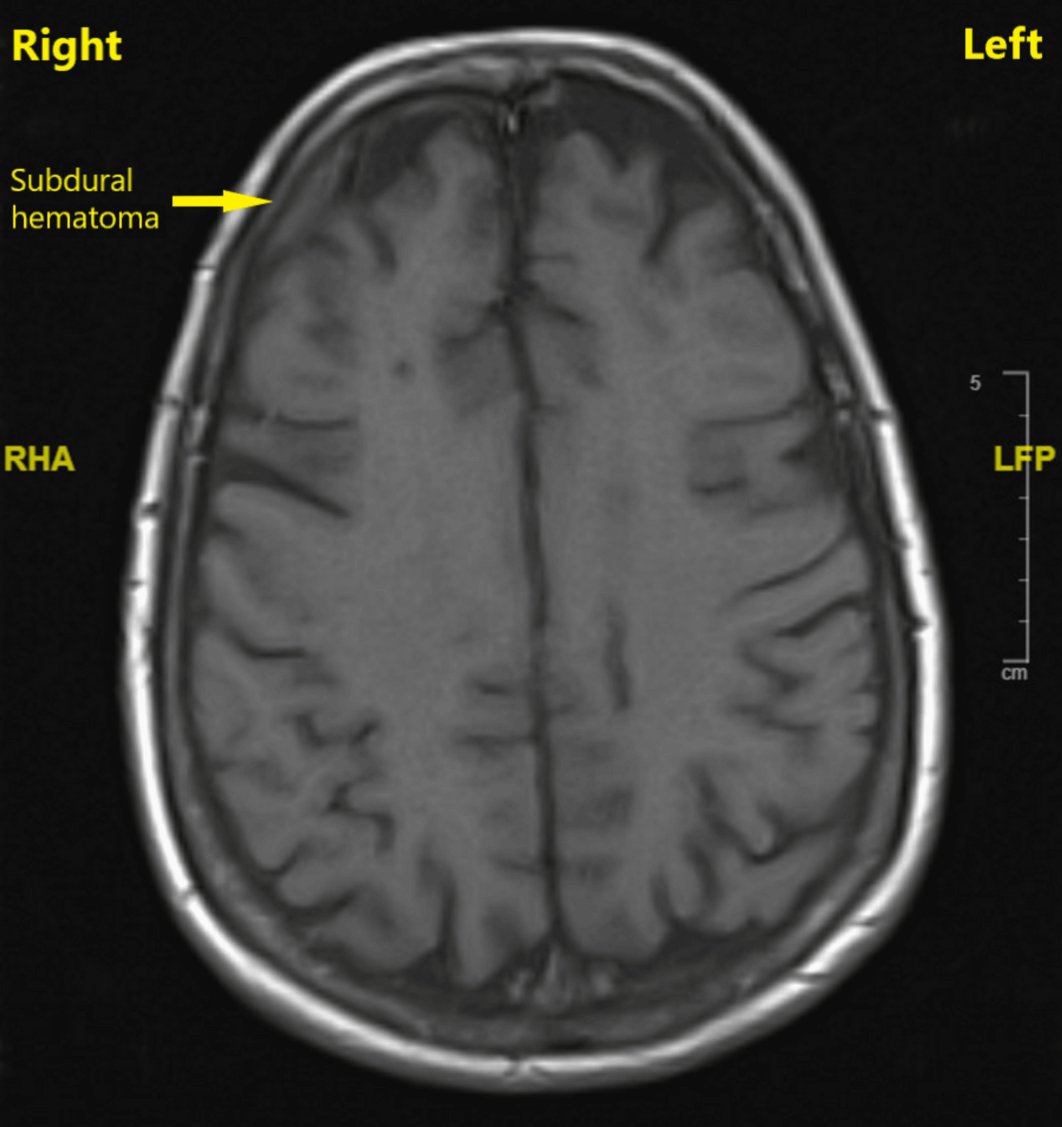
Magnetic resonance imaging of the head 6 hours later with subdural hematoma overlying the right frontal lobe without any evidence of midline shift and without any signs of intracranial hypotension Magnetic resonance imaging of the head performed 6 hours after the initial computerized tomography imaging that detected a subdural hematoma, showing that the hematoma was stable without any midline shift, mass effect, or intracranial hypotension. RHA, right head anterior; LFP, low-frequency potential.
